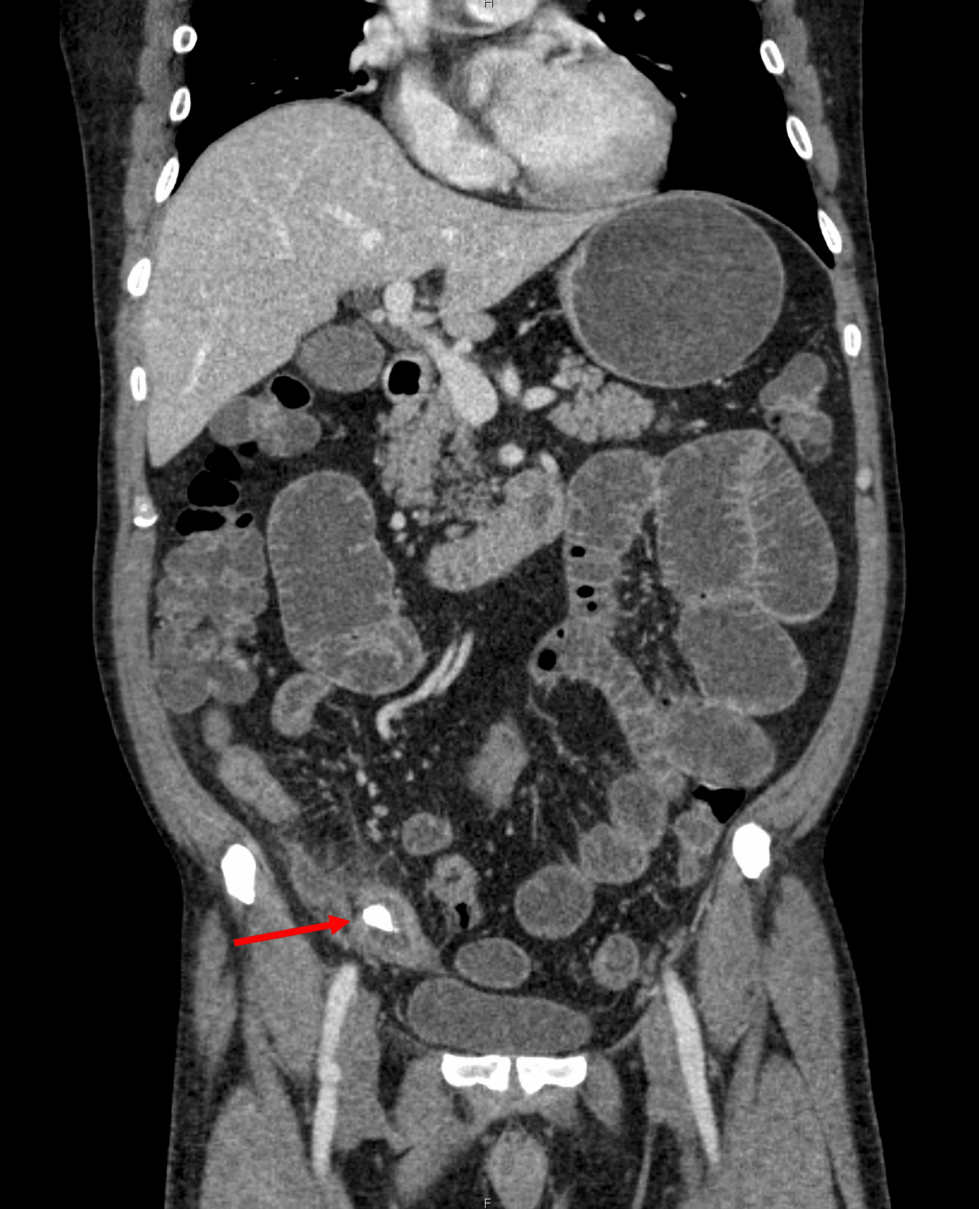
Patient’s computed tomography revealing a foreign body (red arrow) in the ileum with proximal small bowel dilation.
